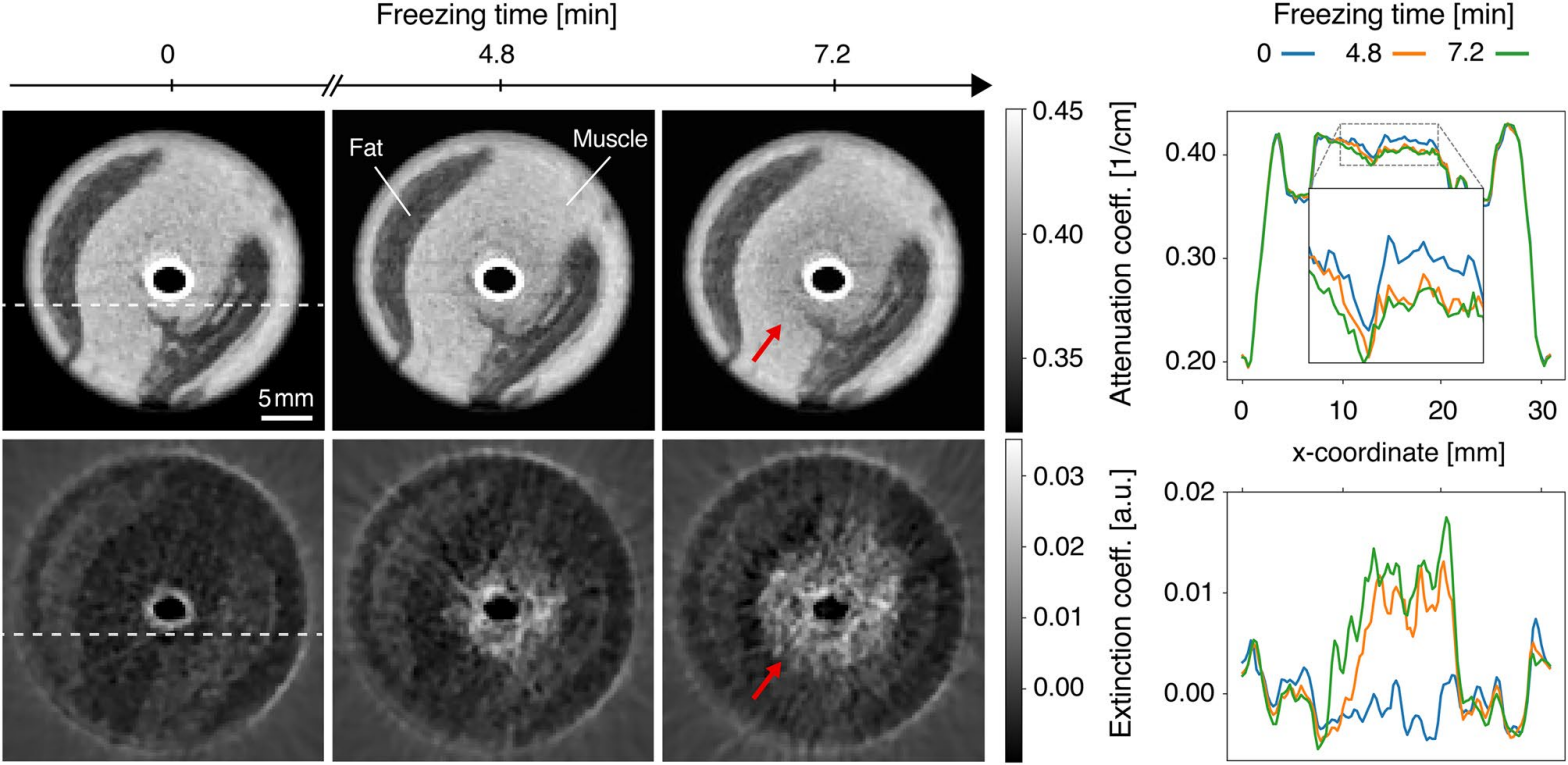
Series of axial slices (left) and line plots (right) near the top of the sample in dark-field and attenuation images at different time points. A radially symmetric increase in signal strength is observed in the dark-field while the same region appears slightly darker in the attenuation images. The red arrows mark the boundary of the frozen region, which is easier to see in dark-field. The acquisition time for each multimodal CT scan is 2.4 min. The line plots show the intensity along the dotted white line, averaged over 10 axial slices extending beyond the depicted image plane.
